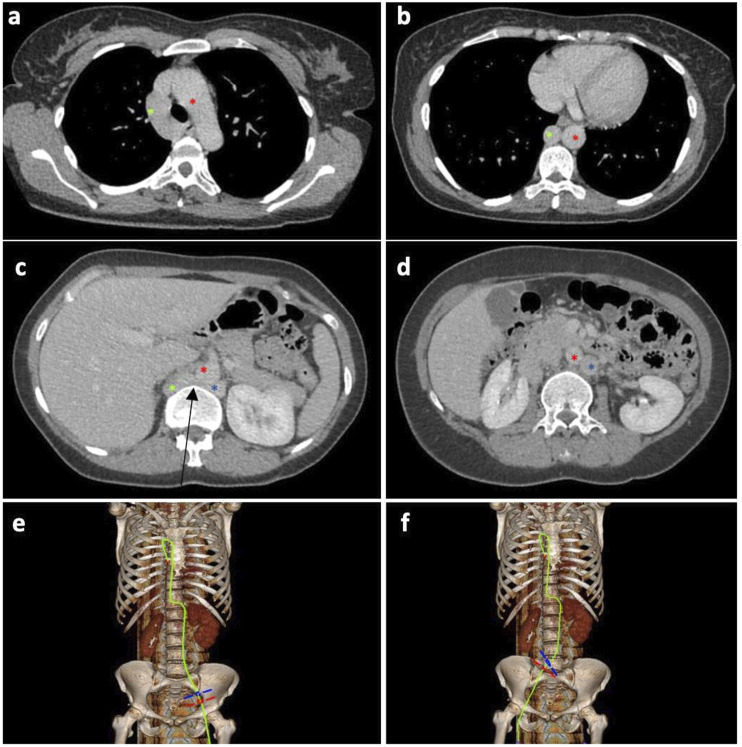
(A-D) Axial contrast enhanced CT displaying azygos continuation (green asterisk) of the left IVC (blue asterisk) with compression of the left renal vein (black arrow) between the spine and aorta (red asterisk). 2(E-F) 3-D reconstruction of the course of venous drainage from the bilateral lower extremities.
